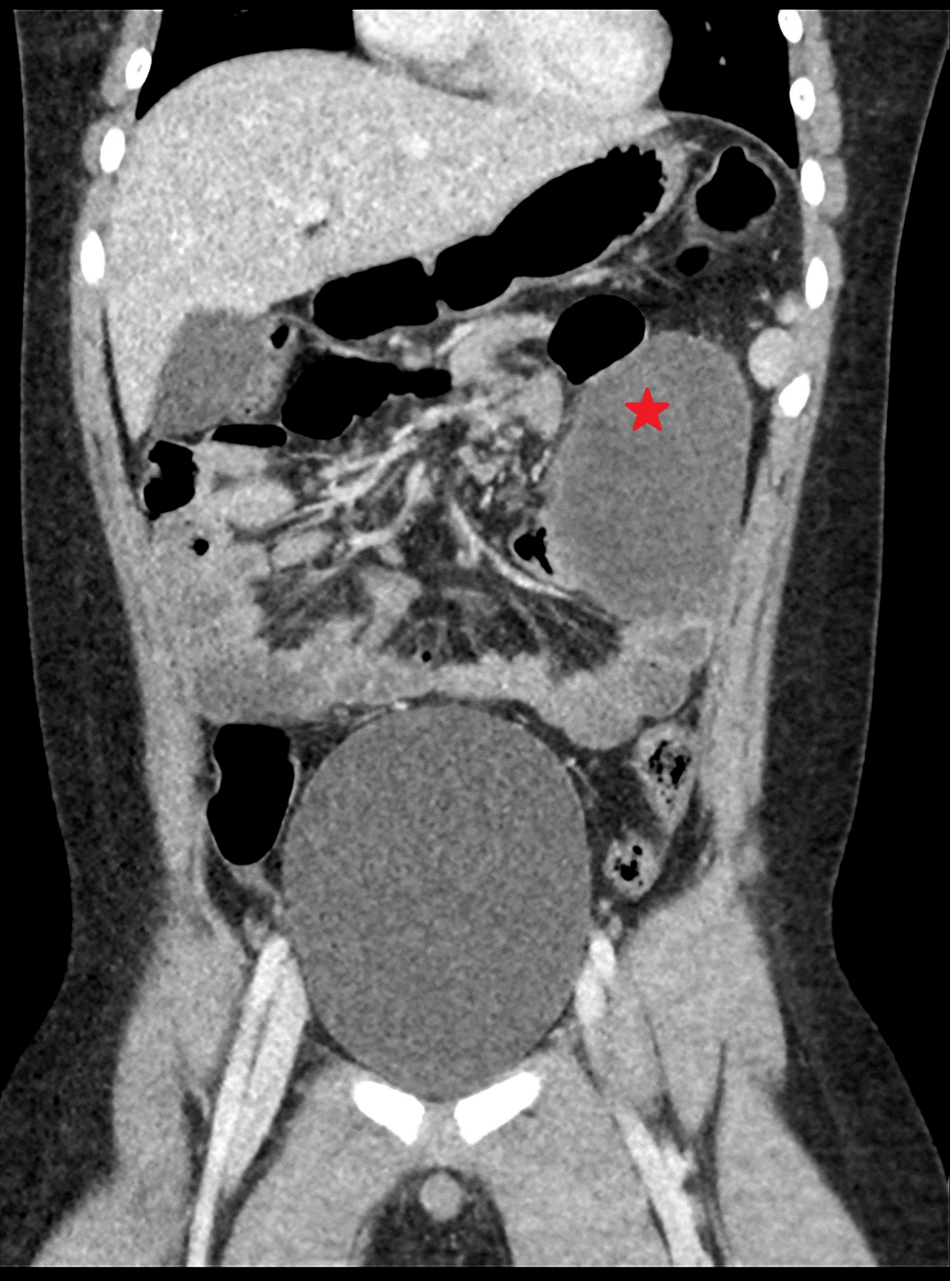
CT Scan - Abdomen Coronal CT showing upper abdominal small intestinal loop dilatation which is more prominent on the left side (red star), measuring about 48 mm in diameter and containing a single air-fluid level.
